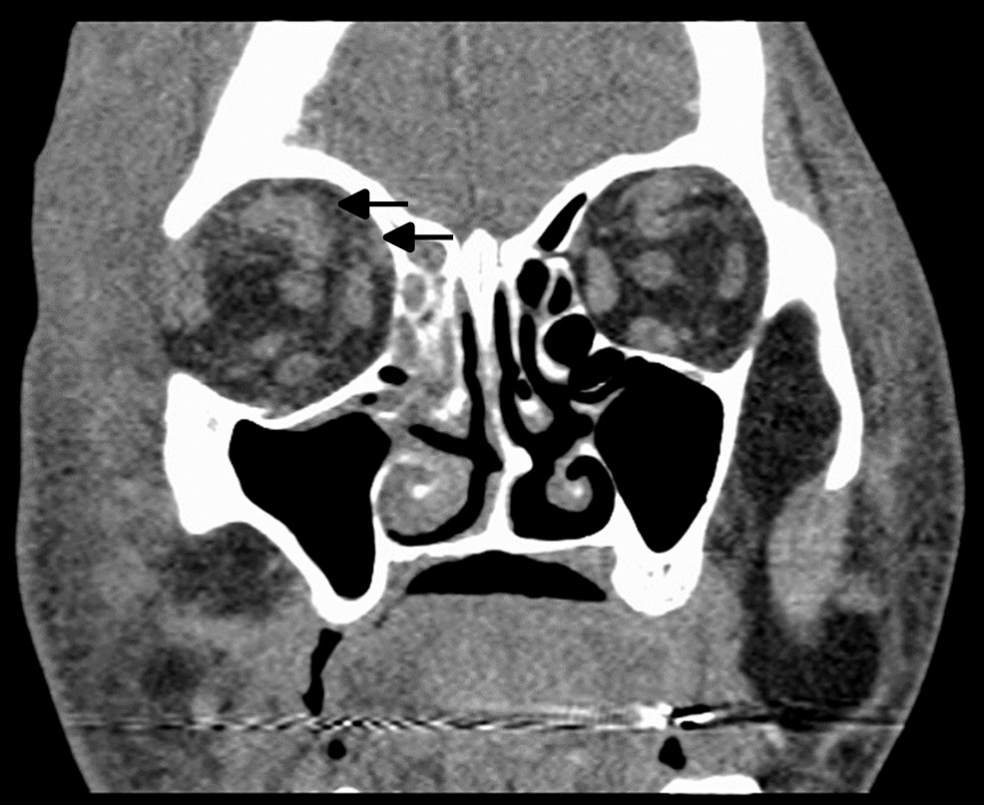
Computed tomography of the orbit CT of the orbits demonstrating stranding and infiltration of the intraorbital fat surrounding the right superior rectus/superior ophthalmic vein and medial to the medial rectus suspicious for orbital cellulitis (black arrows). Note the facial cellulitis and that the right is greater than the left. CT: computed tomography
